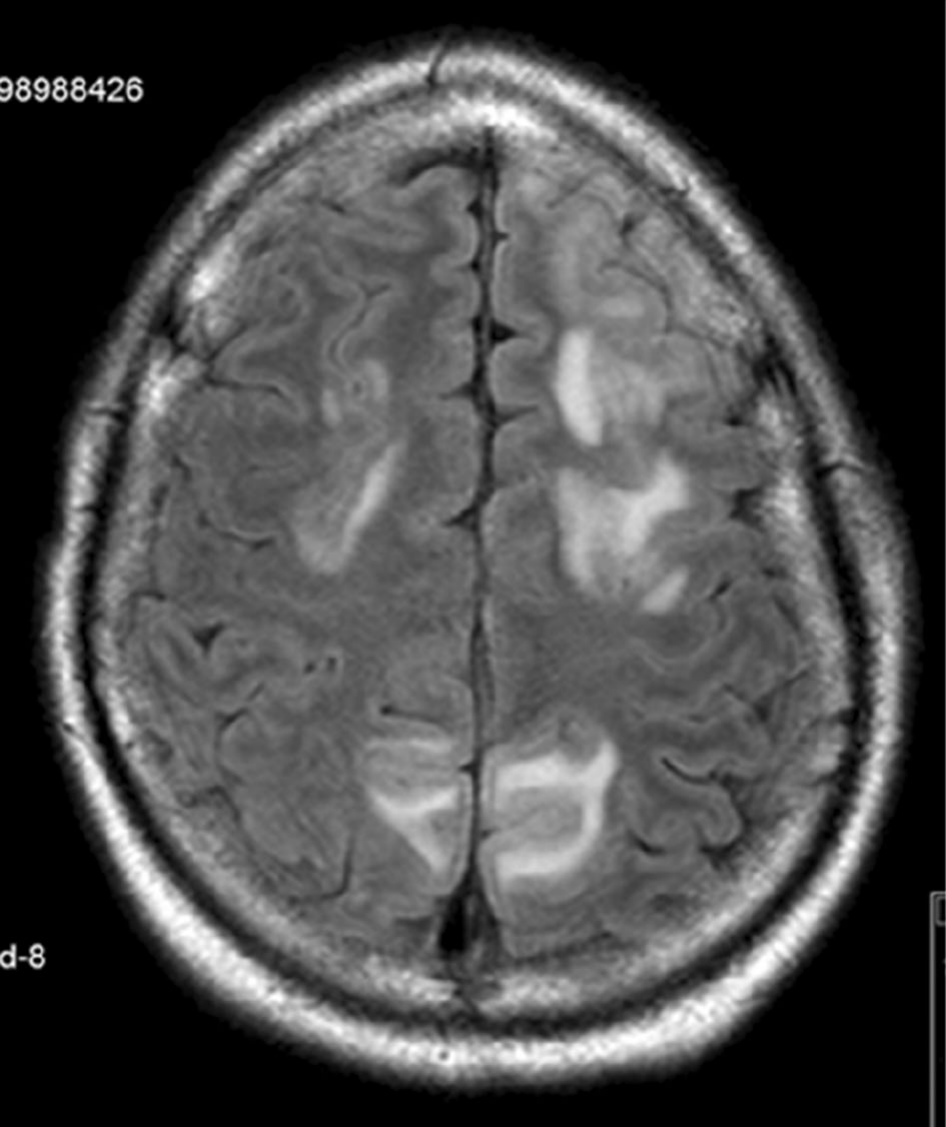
Posterior reversible encephalopathy syndrome in a young patient with T-cell lymphoblastic lymphoma, presenting with headache and seizure after first chemotherapy cycle (pegylated l-asparaginase, vincristine, doxorubicin, and methotrexate): bilateral asymmetrical parieto-frontal hyperintensities on FLAIR sequence
